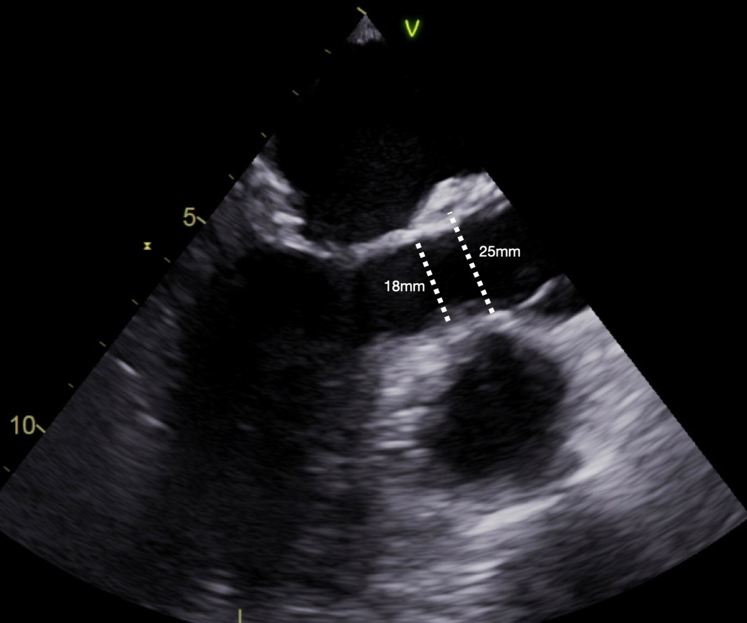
Transesophageal echocardiography, postoperative long-axis view showing the aortic valve and left ventricular outflow tract (in systole) and its enlarged diameters.
